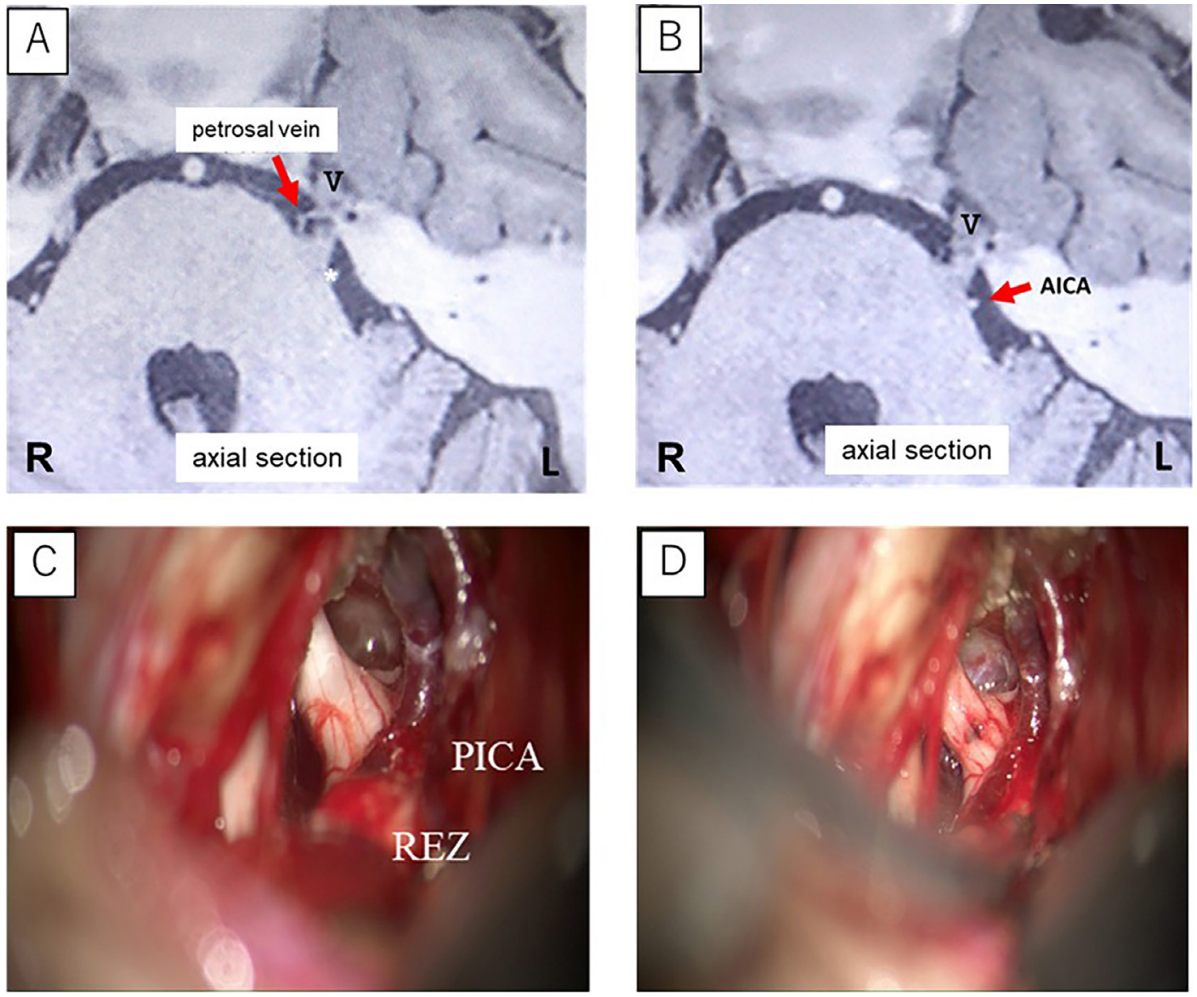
Case 1: (A) Preoperative cranial magnetic resonance imaging (MRI) shows the petrosal vein is not in contact with the trigeminal nerve root. (B) The anterior inferior cerebellar artery (AICA) does not contact the trigeminal nerve root. (C) Intraoperative findings reveal the posterior inferior cerebellar artery (PICA); however, no arterial or venous structures are in contact with the trigeminal nerve root. (D) The trigeminal nerve after nerve combing.
